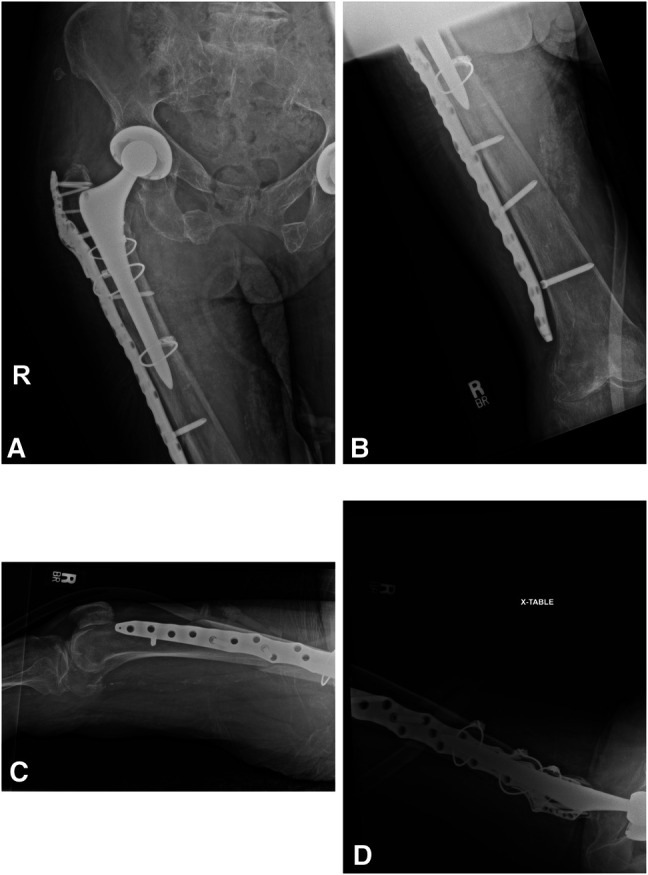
A and B, AP and (C and D) lateral radiographs obtained approximately 11 weeks postoperatively at the time of patient re-presentation to the emergency department for evaluation of right lower extremity pain after a fall. There is an acute supracondylar distal femur fracture as well as evidence of the most distal screw having fretted through the plate.
